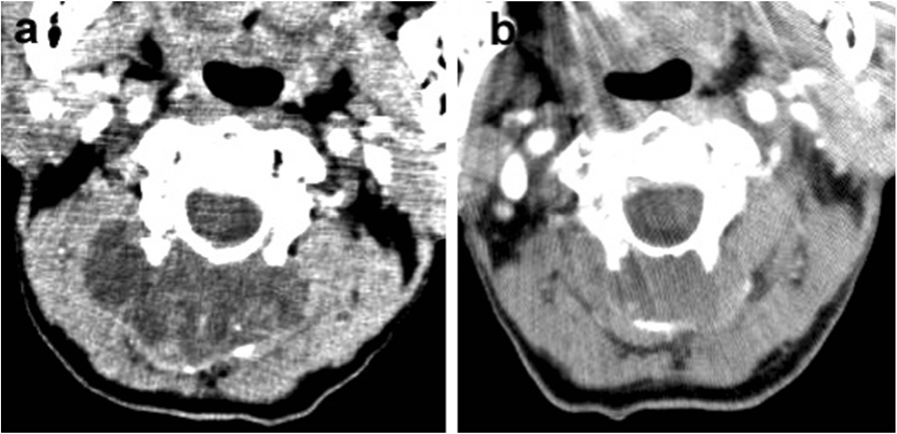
The axial computed tomography (CT) image (a) demonstrated a soft-tissue mass with an amorphous ‘rings and arcs’ calcified matrix and destruction of the C2 lamina. The tumor at the C2 lamina was also observed in a CT image taken at two years before presentation (b) and the tumor was noted to have expanded during the follow up.
